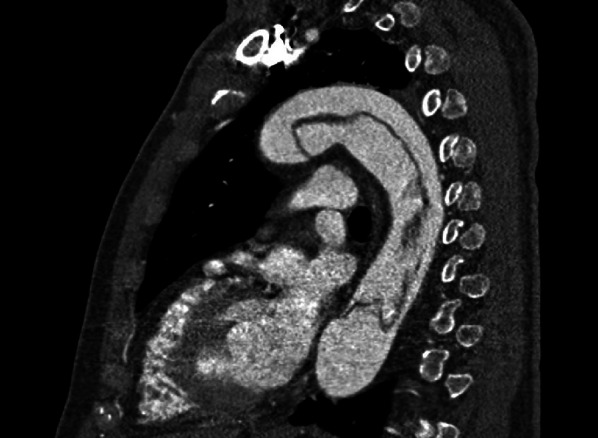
Preoperative sagittal-oblique CT angiography of the chest demonstrating acute type A aortic dissection with a primary intimal tear at the level of the aortic arch extending down in the thoracoabdominal aorta
